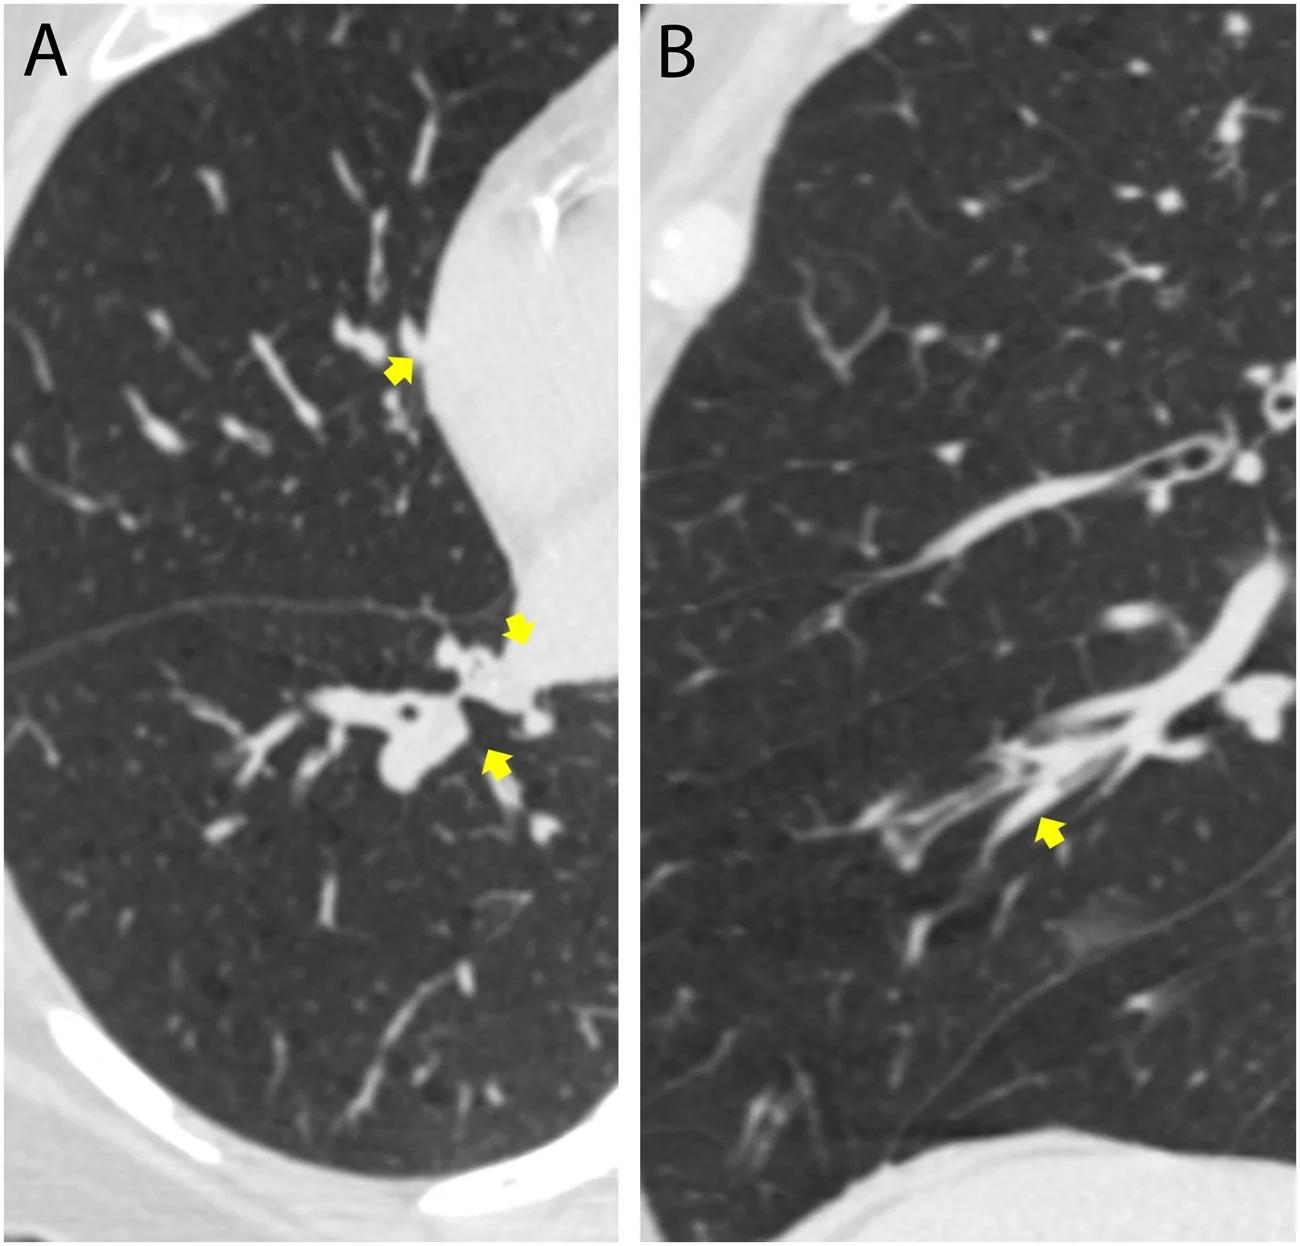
Computed tomography (CT)–detected mucus plugs. Airway-occluding mucus plugs (arrows) on CT images. (A) Axial section of the right middle lobe and right lower lobe. (B) Sagittal section of the right middle lobe of the same individual. Image courtesy of the research team of Dr. Alejandro Diaz.
